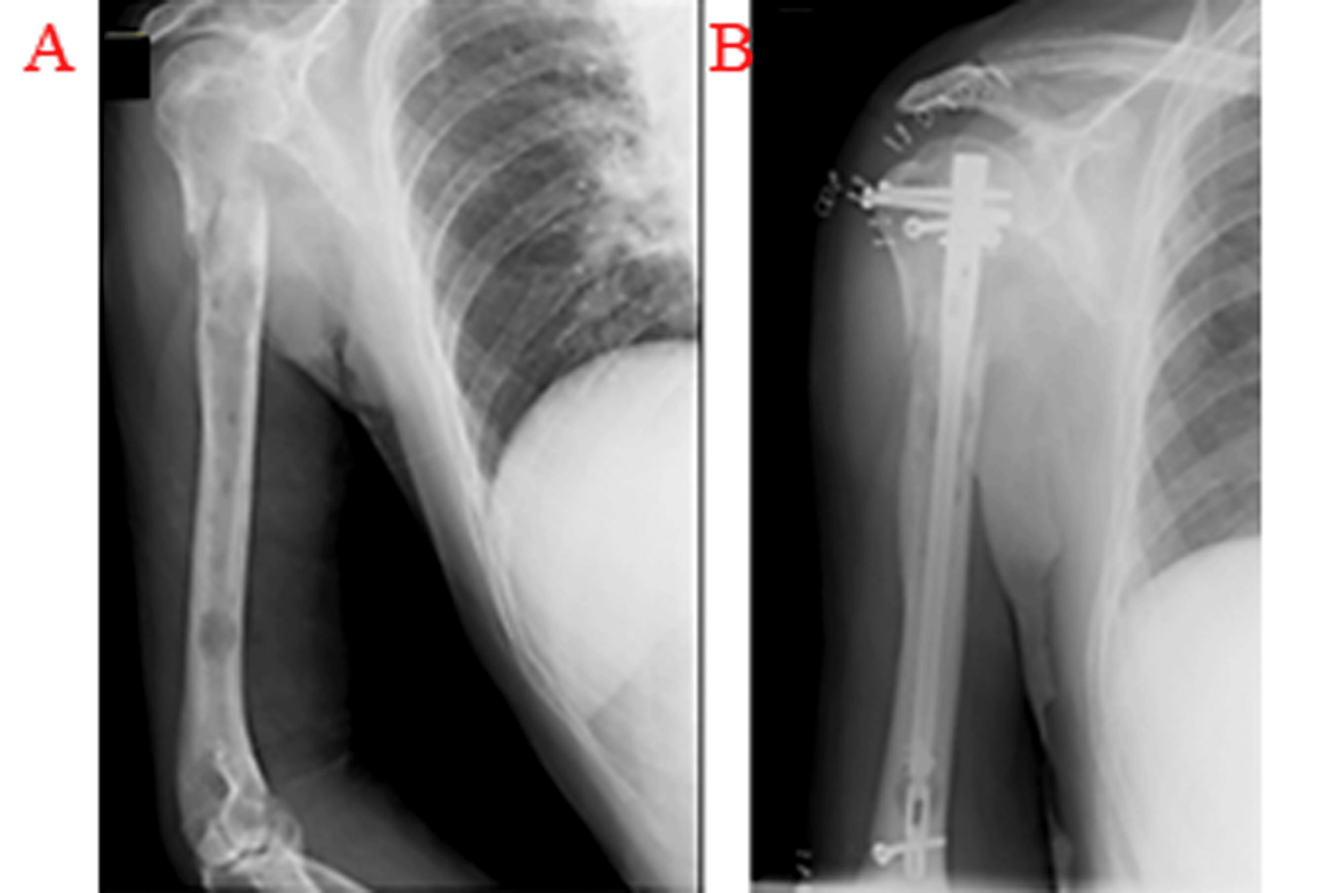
Radiographic findings of a pathological fracture of the right humerus. (A) The primary lesion was lung cancer with osteolysis. (B) Radiographic findings after treatment with IMN. IMN: intramedullary nail.
